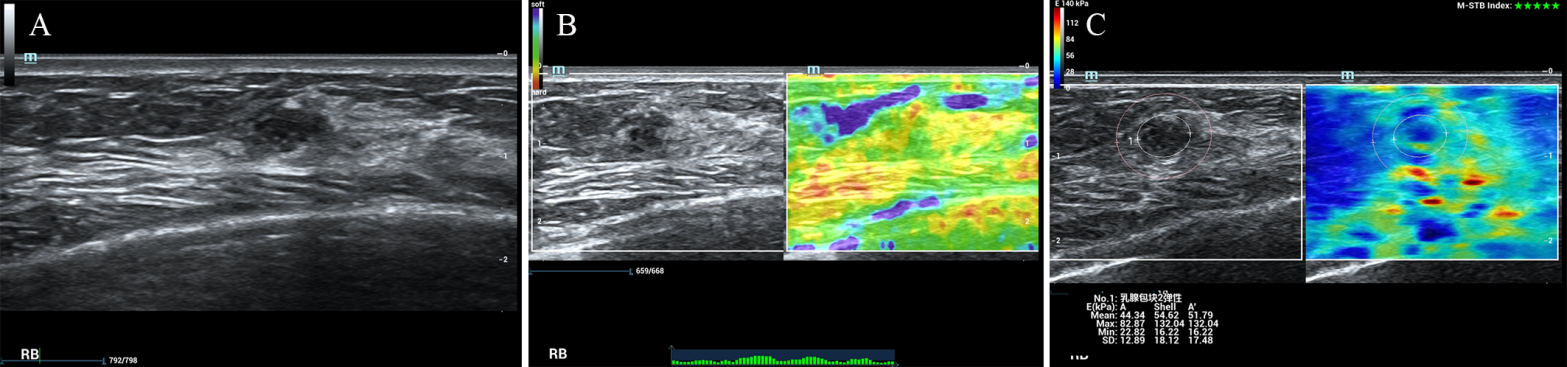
The breast cancer was downgraded by the ES of 1-3 points. A 47-year-old female patient had a lesion on her right breast. Pathologically confirmed middle-grade ductal carcinoma in situ. The size of the lesion was 9mm and ultrasound images of the breast lesion were evaluated as BI-RADS 4a (A), with an elasticity score of 3 (B), Emax-A of 82.9kpa and Emax-A’-S2.5 of 132.0kpa (C).
